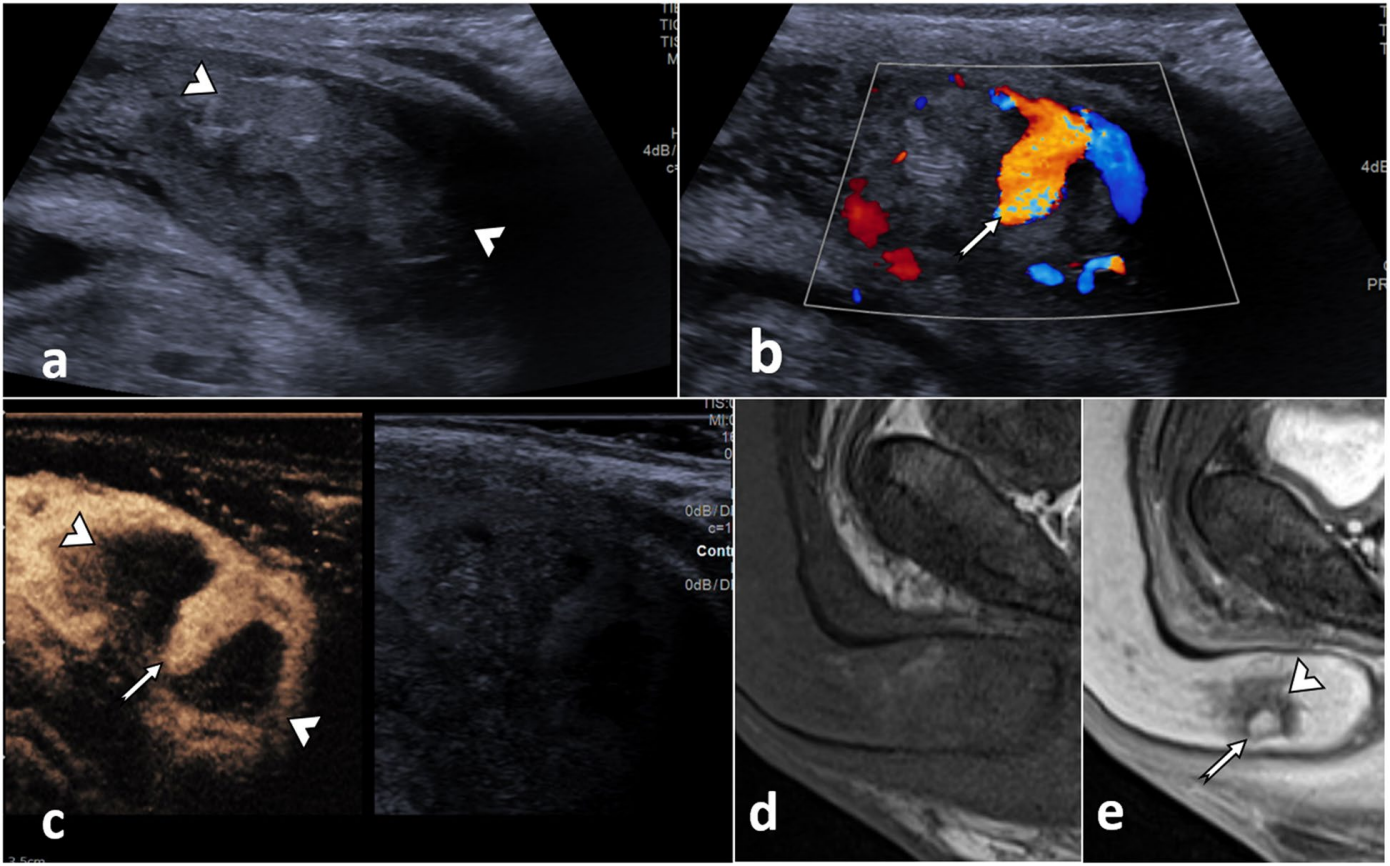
Penile haematoma with pseudoaneurysm. A 38-year-old man presented to the emergency department following a pedal bicycle accident with blunt trauma to the non-erect penis, with clinical high-flow priapism at presentation. Ultrasound was performed. In a sagittal plane in B mode (a) where an ill-defined area of mixed echogenicity (between arrowheads) in the right corpus cavernosum at the base of the penis, suspicious of haematoma is seen. The colour Doppler US (b) shows a deformity of a branch of the cavernosal artery (arrow) with turbulent flow in its interior, consistent with pseudoaneurysm. CEUS (c) confirms the presence of the non-enhancing haematoma (between arrowheads) and the pseudoaneurysm, which enhances avidly (thin arrow) in the arterial phase. A complementary MRI was performed (sagittal T1 pre-contrast (d), sagittal T1 FSE C+ (e)), showing an area of hyperenhancement of the right corpus cavernosum (arrowhead), compatible with the known haematoma. Within the haematoma, there is a hyperenhancing saccular area corresponding to the pseudoaneurysm (arrow). Note the higher spatial resolution of US compared to MRI in assessing the penis
